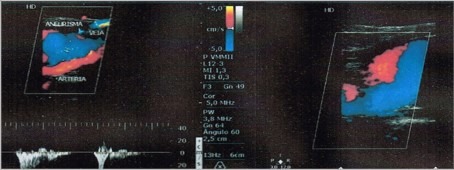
Doppler ultrasonography showing the popliteal vein aneurysm.
